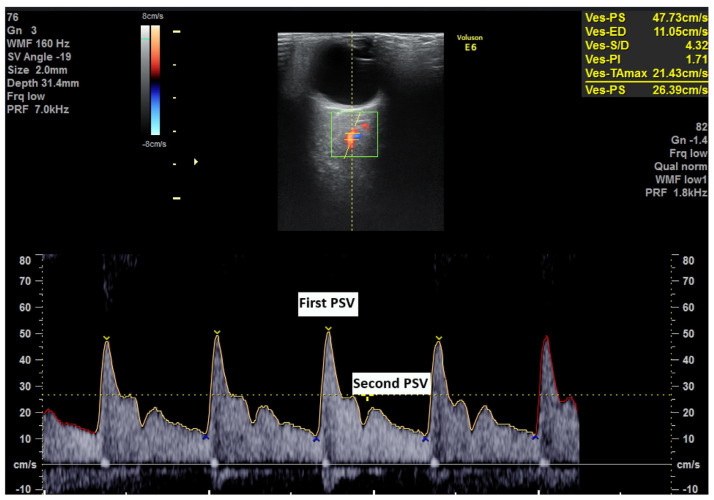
Ophthalmic artery Doppler with indices.
